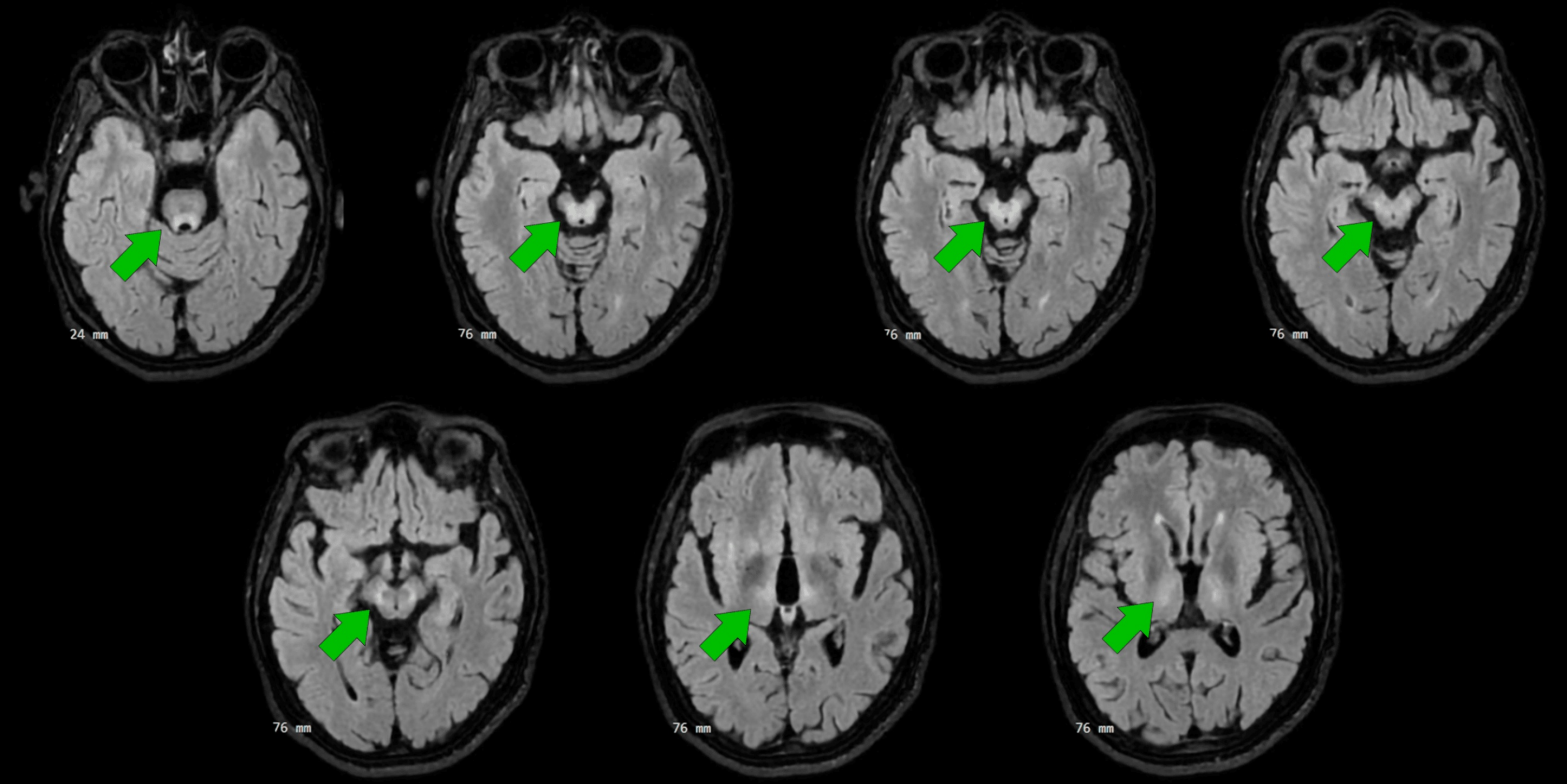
Fluid-attenuated inversion recovery (FLAIR) sequence cuts of brain magnetic resonance imaging showing bilateral symmetric hyperintensity of the anterior and middle thalami, extending through the cerebral peduncles into the pons and floor of the fourth ventricle
